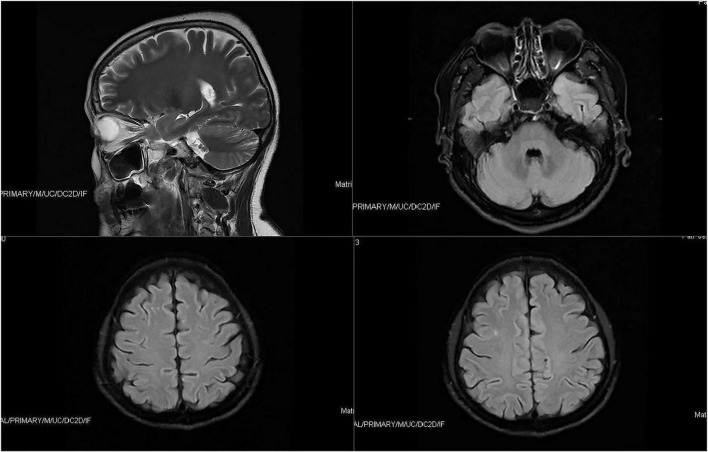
Brain magnetic resonance imaging only showed a few ischemic foci in the brain parenchyma and paranasal sinusitis.
